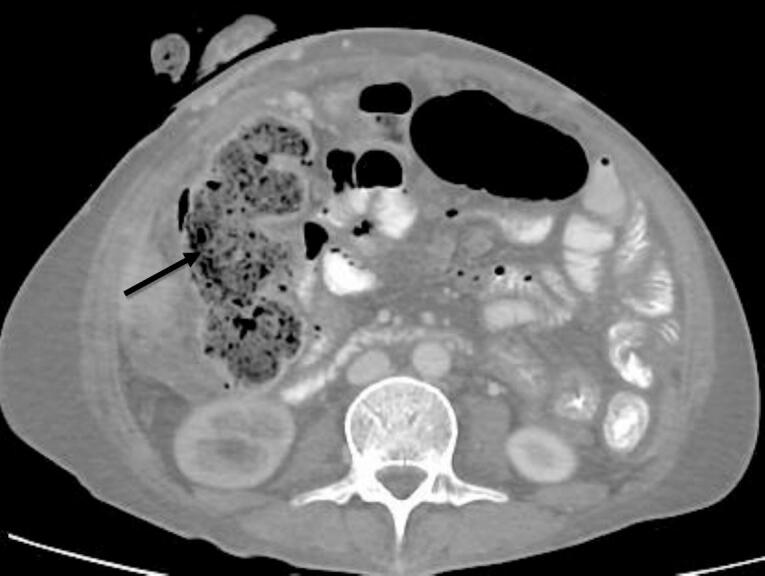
Axial CT. Abdominal CT scan with IV and oral contrast - venous phase, axial view: the colon appears loaded colon (black arrow). Incidentally we found focal aneurysmal dilatation of the celiac trunk measures about 1.3 cm in diameter with evidence of internal dissecting flap.
